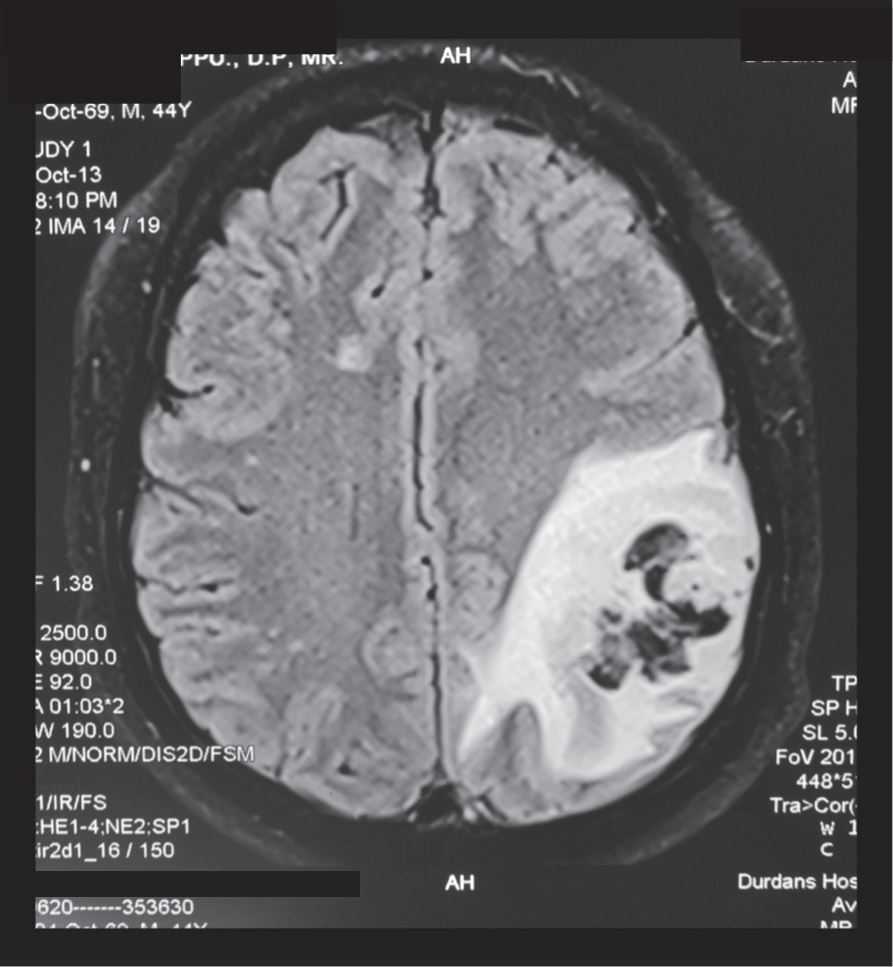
Fluid-attenuated inversion recovery magnetic resonance imaging of brain showing an acute left parieto-occipital infarct with haemorrhagic transformation and perilesional oedema.
